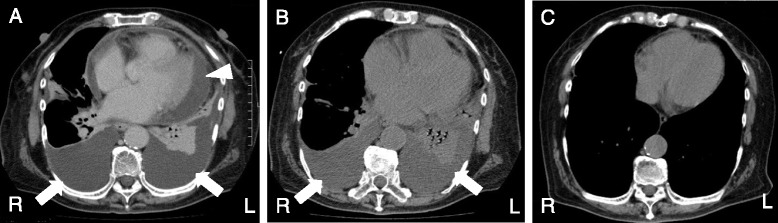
Chest computed tomography. a Chest computed tomography on the day before steroid therapy showed moderate bilateral pleural (*arrows*) and pericardial effusions (*arrowhead*). b Chest computed tomography after steroid therapy showed that the pleural effusion had decreased (*arrows*) and the pericardial effusion had disappeared. c Follow-up chest computed tomography on day 38 showed no pleural or pericardial effusions
